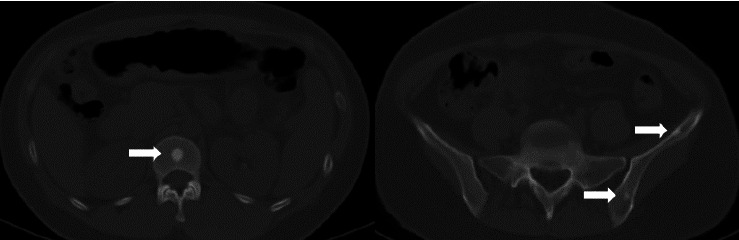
Four-year follow-up CT imaging of an osteoma involving the left left ilium and thoracic vertebral bone.
